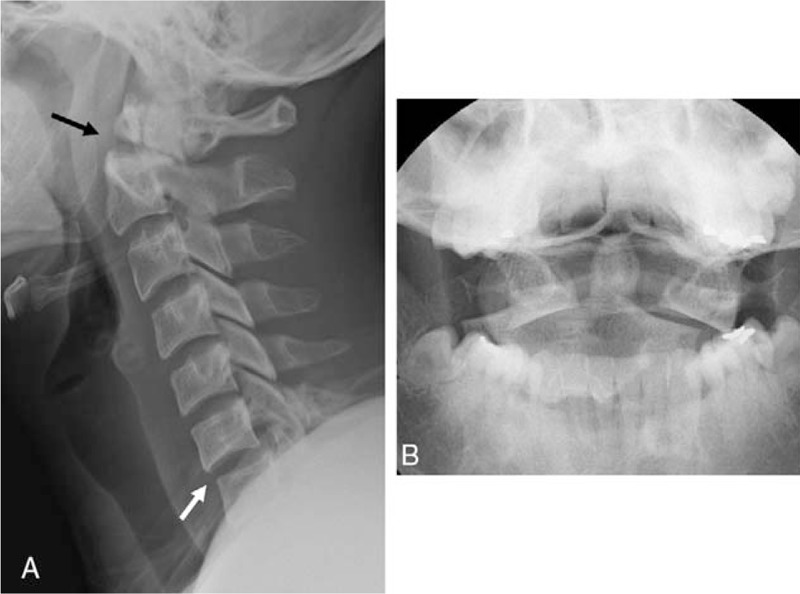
Lateral radiograph (A) and open mouth view (B) showing a posterolateral dislocation of C1 on C2 with posterolateral displacement of the proximal fragment of the dens (black arrow) (type II fracture of Anderson and D’Alonzo classification) and anterior subluxation of C6–7 (white arrow).
